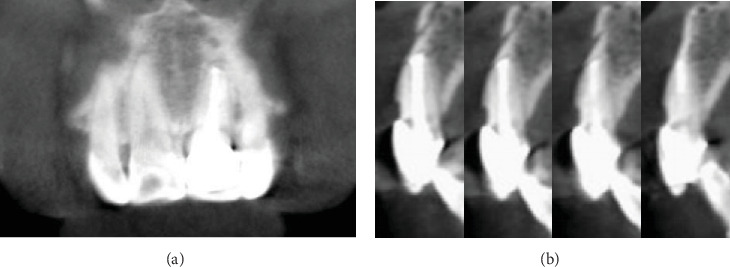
CBCT radiographic evaluation at the 12-month follow-up. (a) Coronal and (b) sagittal images showed the resolution of the low-density lesion at the apex.
